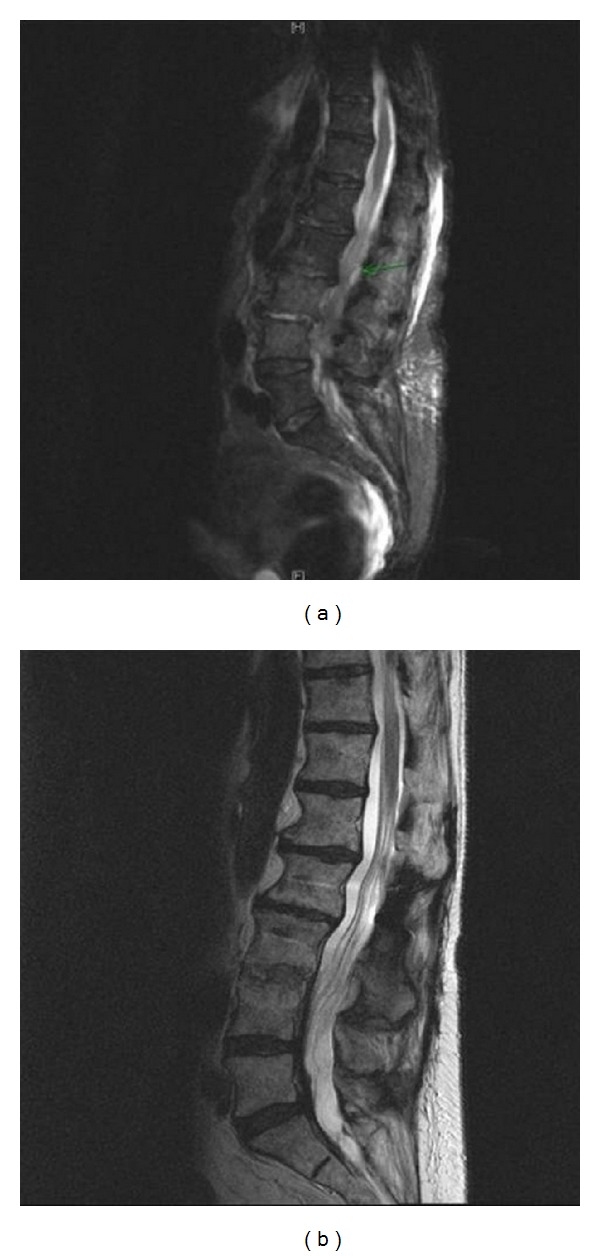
(a) Preoperative MR images of a 75-year-old female patient with a L3/4 MRSA-spondylodiscitis and intraspinal abscess (“green arrow”); (b) 18 months after surgery, a bony fusion with no signs of any infection is evident.
